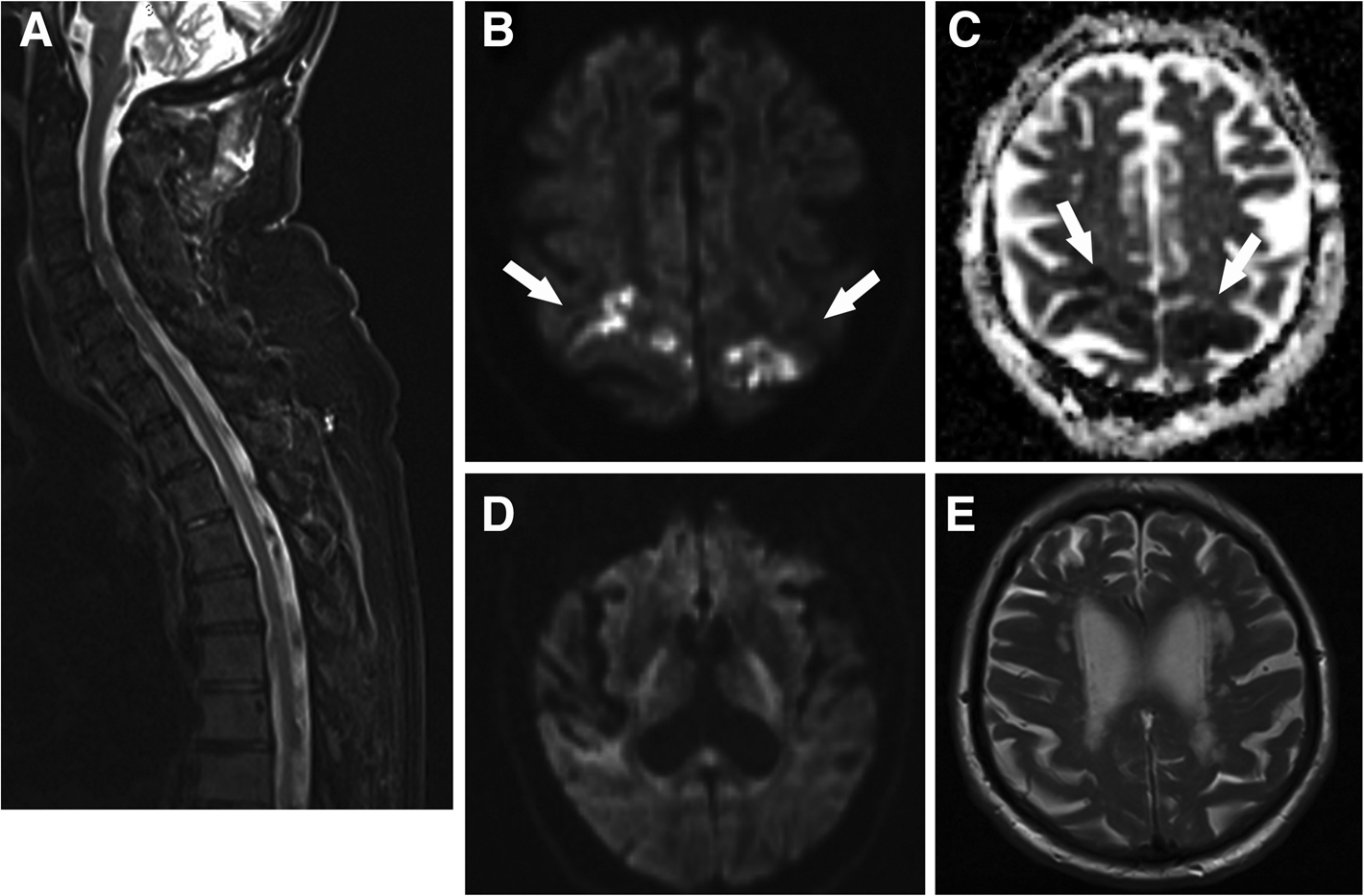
Bilateral acute ischemia in the motor cortex area. (A) Spinal cord magnetic resonance imaging (MRI) was normal and showed no signs of compression or ischemia. Of note, acute infarction, for example of the anterior spinal artery may be undetected in early spinal MRI. (B and C) Diffusion (B) and apparent diffusion coefficient-weighted (C) brain MRI revealed acute infarctions in the precentral gyrus on both sides (medial and parietal parts). (D) At the level of the internal capsule no pathological diffusion restrictions were found. (E) In contrast to diffusion, T2-weighted images did not show any signs of tissue demarcation confirming the acuteness of stroke onset.
